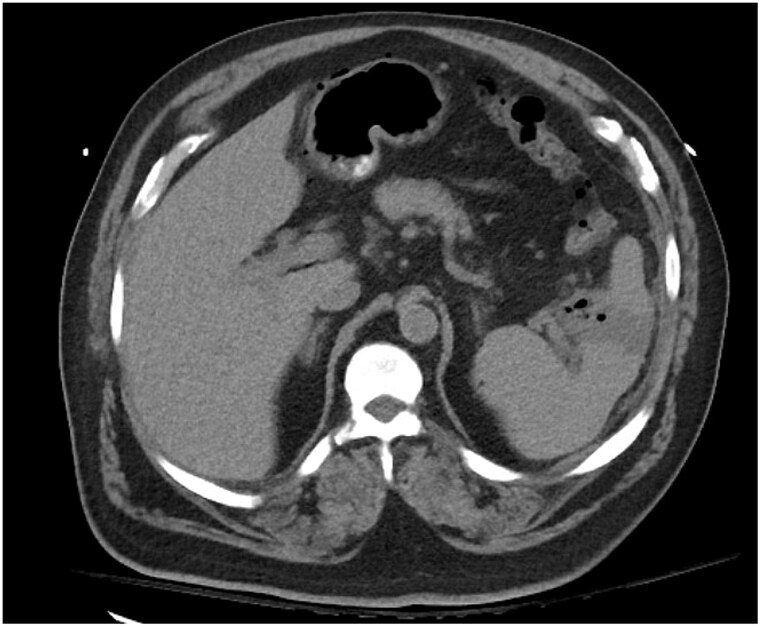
Axial CT section demonstrating the splenic abscess.
